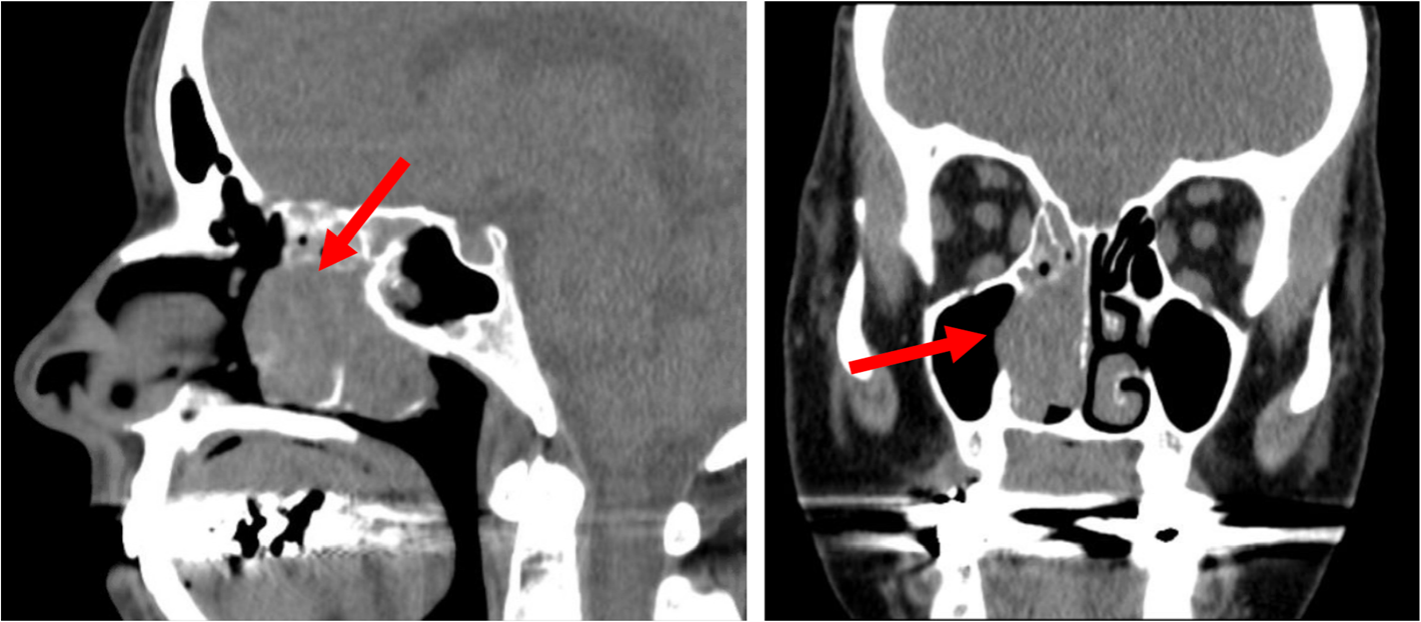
Maxillofacial CT scan of patient in case 1 demonstrated a large polypoid mass in the right middle turbinate (red arrows)
